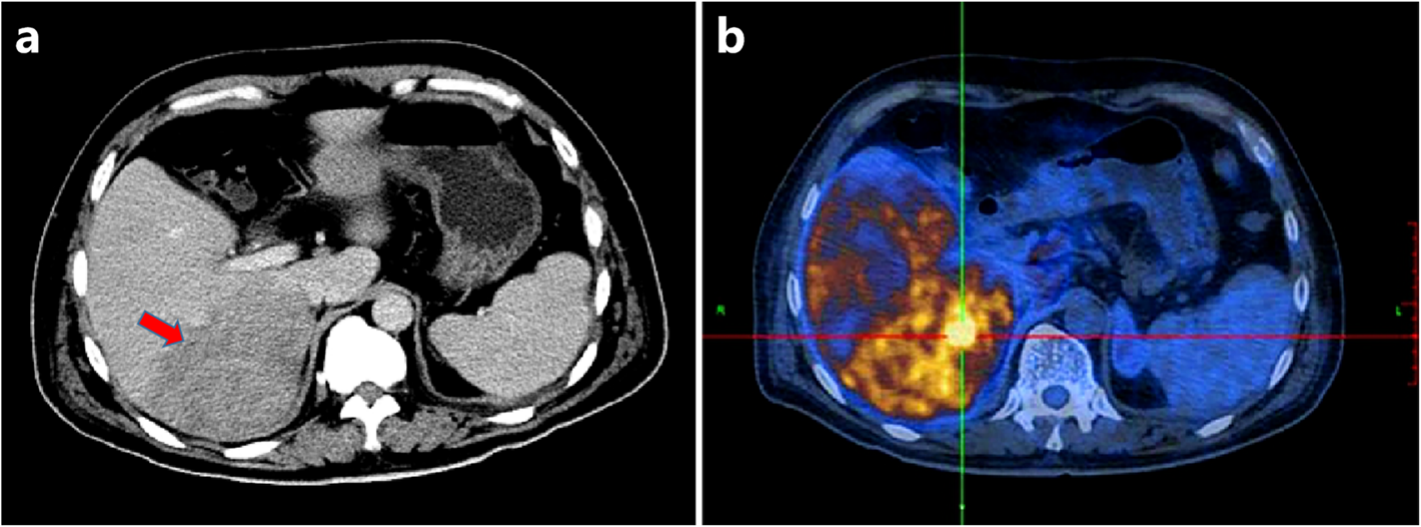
a Abdominal CT showed a slightly lower density lesion of 11.5 × 4.8 cm (arrow) in the right liver lobe with hepatosplenomegaly. b PET-CT revealed abnormal fluorodeoxyglucose uptake in the liver
